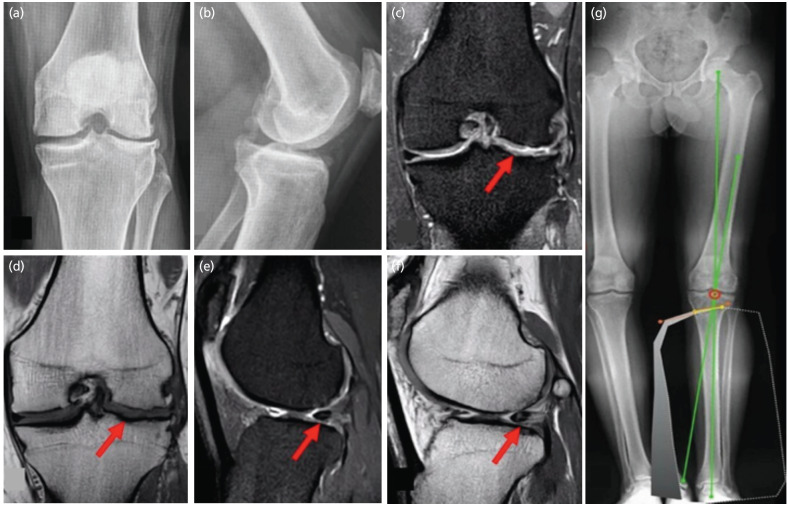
Pre-surgical diagnostic images. (a) Left knee radiograph AP view. (b) Lateral view with Kellgren-Lawrence classification OA grade III in the lateral compartment. (c) In pre-operative magnetic resonance imaging of the left knee, the red arrow indicates the chondral lesion in the lateral tibiofemoral compartment with femoral predominance in axial view with T1 sequence. (d) Fat-suppressed T2-weighted fast spin-echo sequence. (e) The red arrow indicates the medial meniscus posterior horn injury in sagittal view with T1 sequence. (f) Fat-suppressed T2- weighted fast spin-echo sequence. (g) Bilateral genu varum and surgical planning of tibial osteotomy. Left knee: Mechanical axis deviation (MAD) of 19mm, the mechanical lateral distal femoral angle (mLDFA) of 93°, medial proximal tibial angle (MPTA) of 87°, joint line convergence angle of 0°, correction angle of 7° (calculated to 50% of the tibial plateau), open wedge calculated of 6mm.
